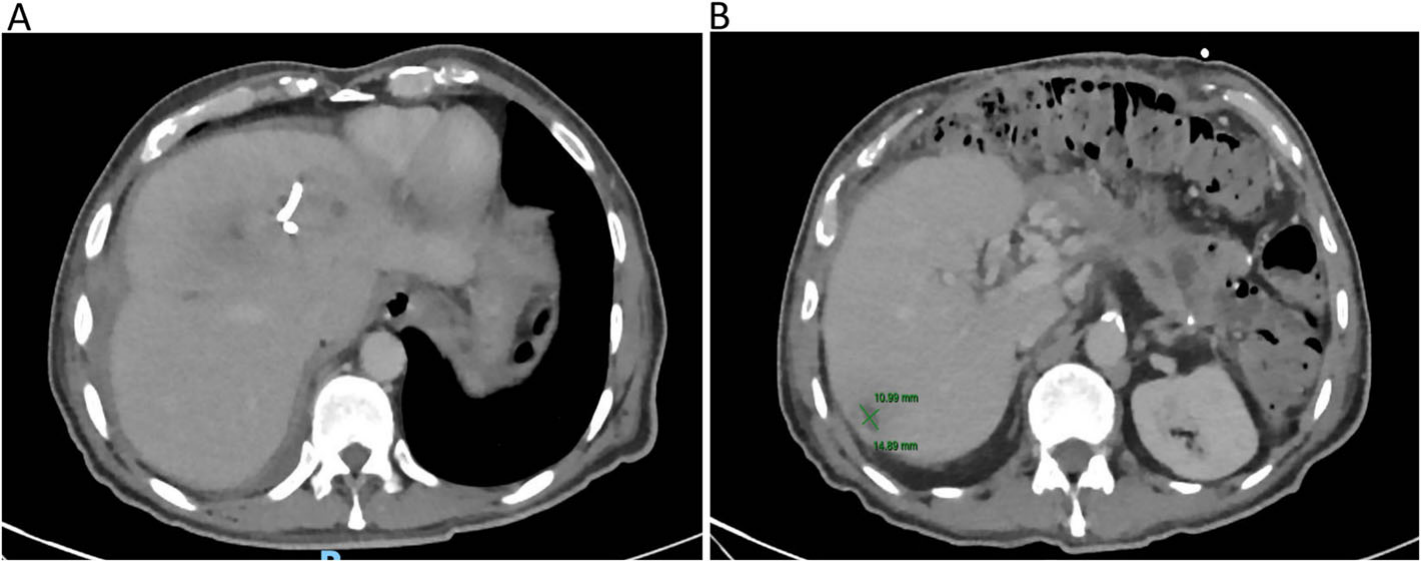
CT scan was taken 26 days after the CT-guided percutaneous drainage was performed. (A) Note the dramatic change in the size of the abscess localized on liver segment VIII. (B) The abscess localized on liver segment VI continued to decrease in size (1.4 cm × 1.0 cm) with antibiotic therapy
